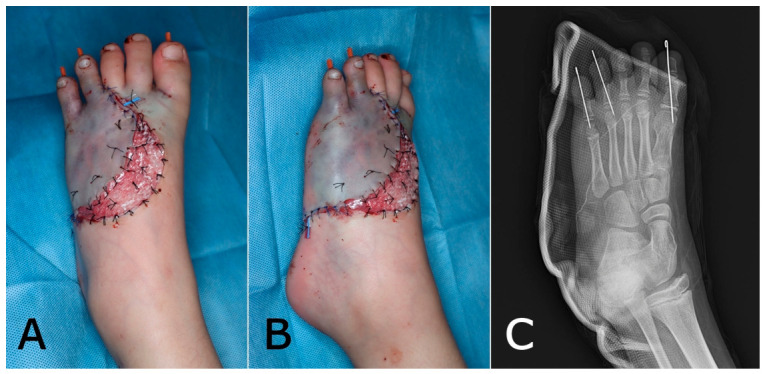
Immediate postoperative clinical photographs and radiograph. (A,B) Immediate postoperative clinical photographs after open reduction, K-wire fixation, debridement, and primary closure of the degloved flap, with artificial dermal grafting using Pelnac applied to the skin defect. (C) Postoperative radiograph confirming reduction and K-wire fixation.
